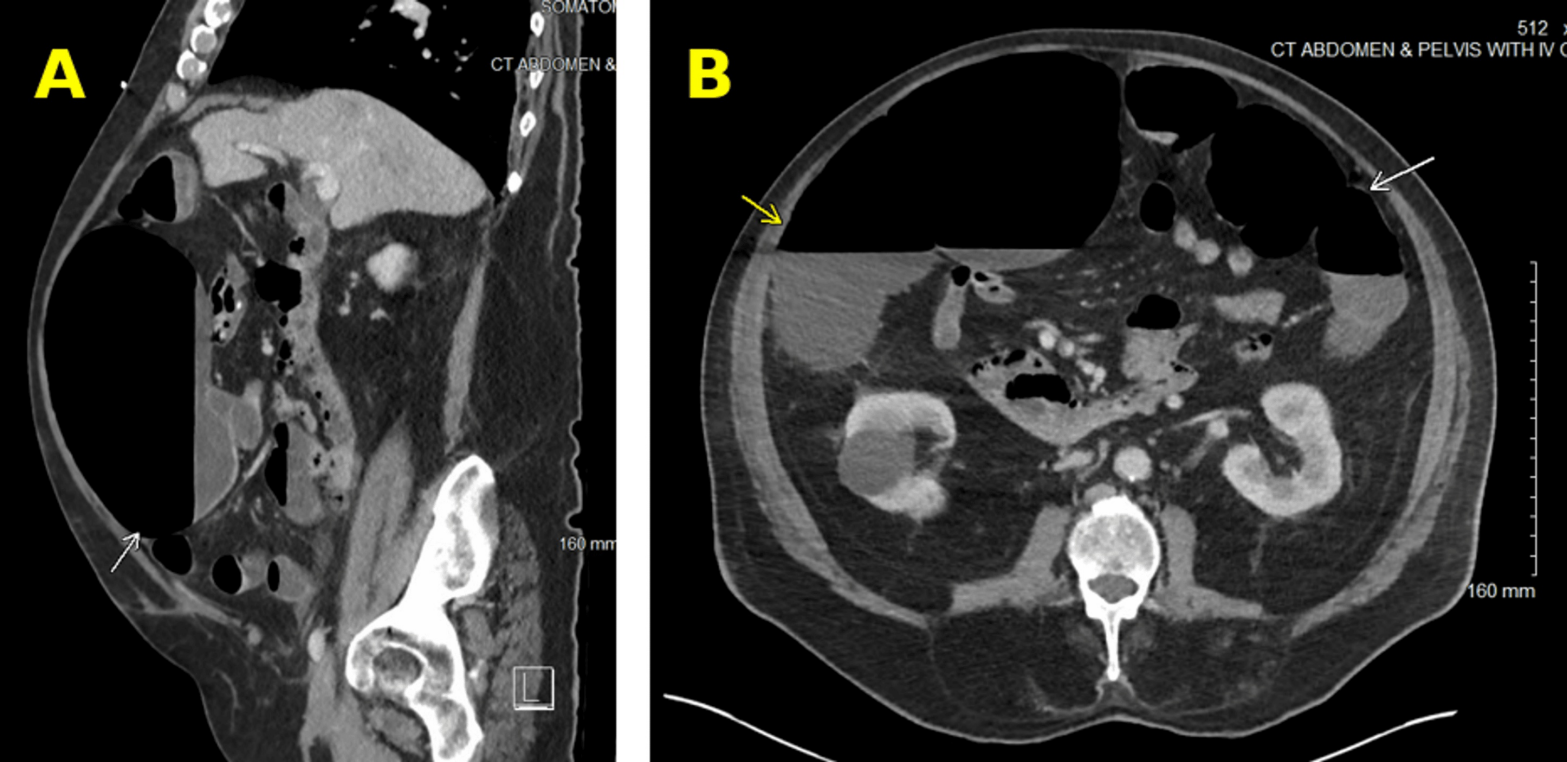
(A) Sagittal view of CT abdomen and pelvis showing cecal dilation (white arrow); (B) Axial view of CT abdomen and pelvis showing cecal dilation (yellow arrow) and fluid levels in the distal colon (white arrow)
